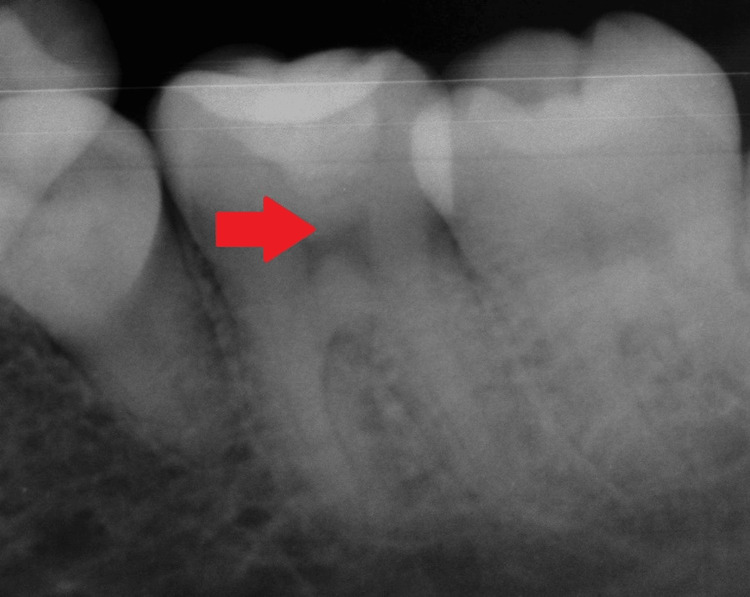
Follow-up intraoral periapical radiograph after one year The arrow shows the formation of the dentin bridge at a one-year follow-up.
